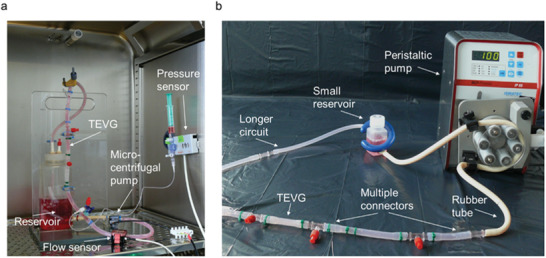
Visual display of the custom‐made bioreactor modifications. a) The closed bioreactor circuit was connected to a 500 mL medium reservoir, a micro‐centrifugal pump, and to pressure and flow sensors. b) Later, the bioreactor circuit was equipped with longer silicon and rubber tubes, a small medium reservoir fitting into the 7T MRI, and a peristaltic pump so that TEVGs were easily insertable and removable during the imaging procedure.
